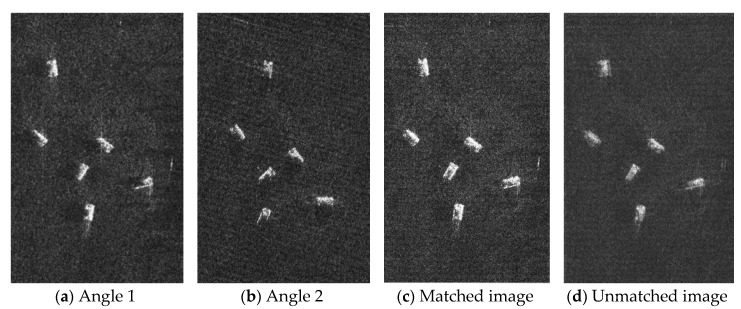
Image fusion results of Range–Doppler algorithm.
